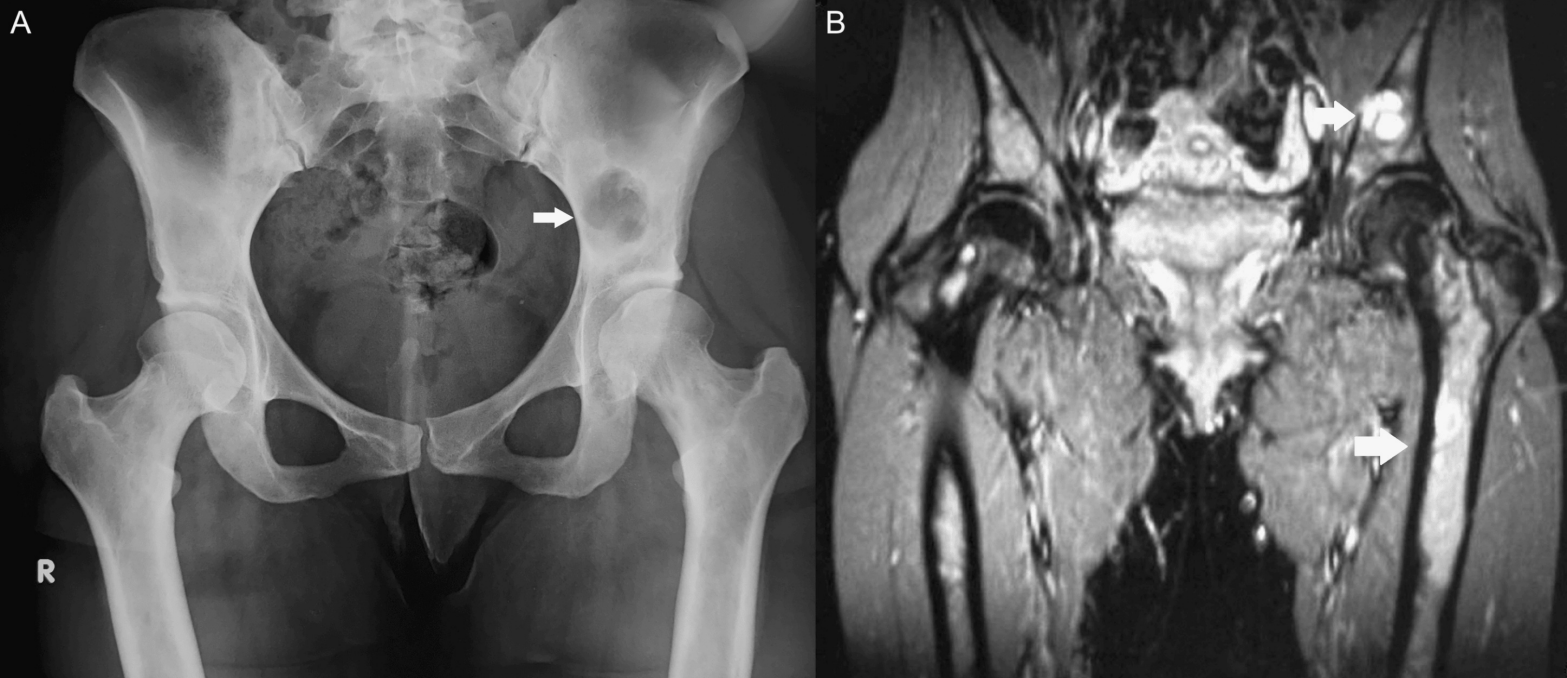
Frontal radiograph (A) and coronal STIR MR image (B) of the pelvis and hips. Shows a prominent FD lesion (arrow) in the left iliac bone, and (B) reveals extensive FD lesions involving the femora and the iliac bones. There is also moderate expansion of bone in the left femur. STIR MRI: Short Tau Inversion Recovery MRI
